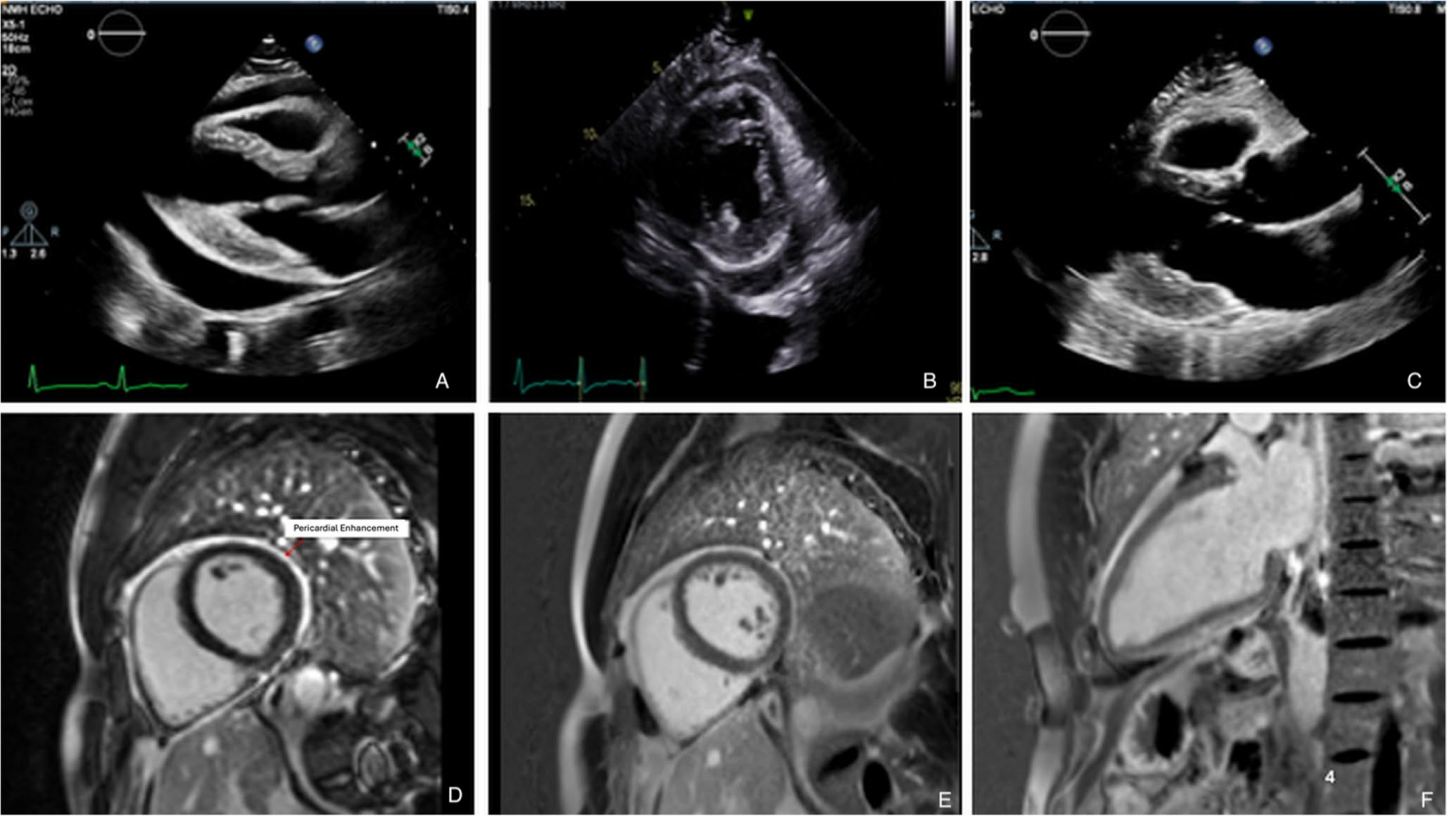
AU: Case example of effusive–constrictive pericarditis with resolution after anti-inflammatory therapy and Rilonacept. Serial transthoracic echocardiography videos demonstrate A pericardial effusion, B persistent constrictive physiology after pericardiocentesis, and C resolution following treatment with NSAIDs, colchicine, prednisone, and subsequent Rilonacept monotherapy. Cardiac magnetic resonance (CMR) images showD pericardial late gadolinium enhancement (LGE) and E, F resolution of pericardial inflammation after 18 months of Rilonacept therapy
